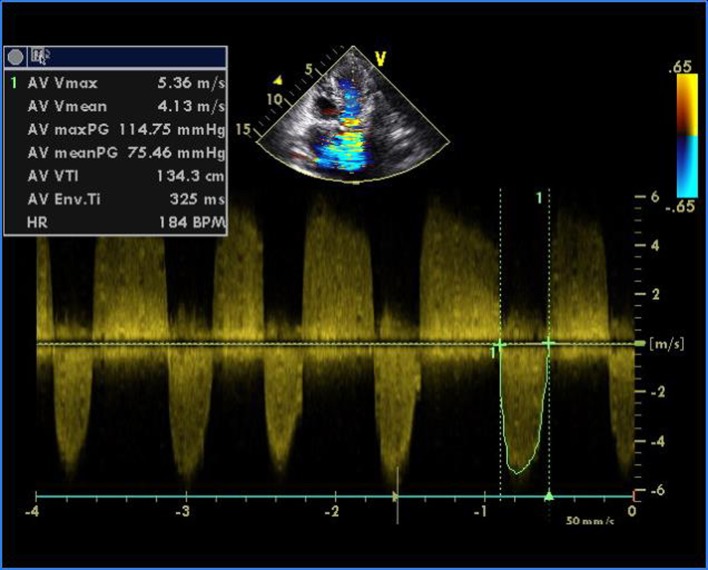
Transthoracic echocardiogram of apical five-chamber view showing severe aortic stenosis and mild regurgitation.
